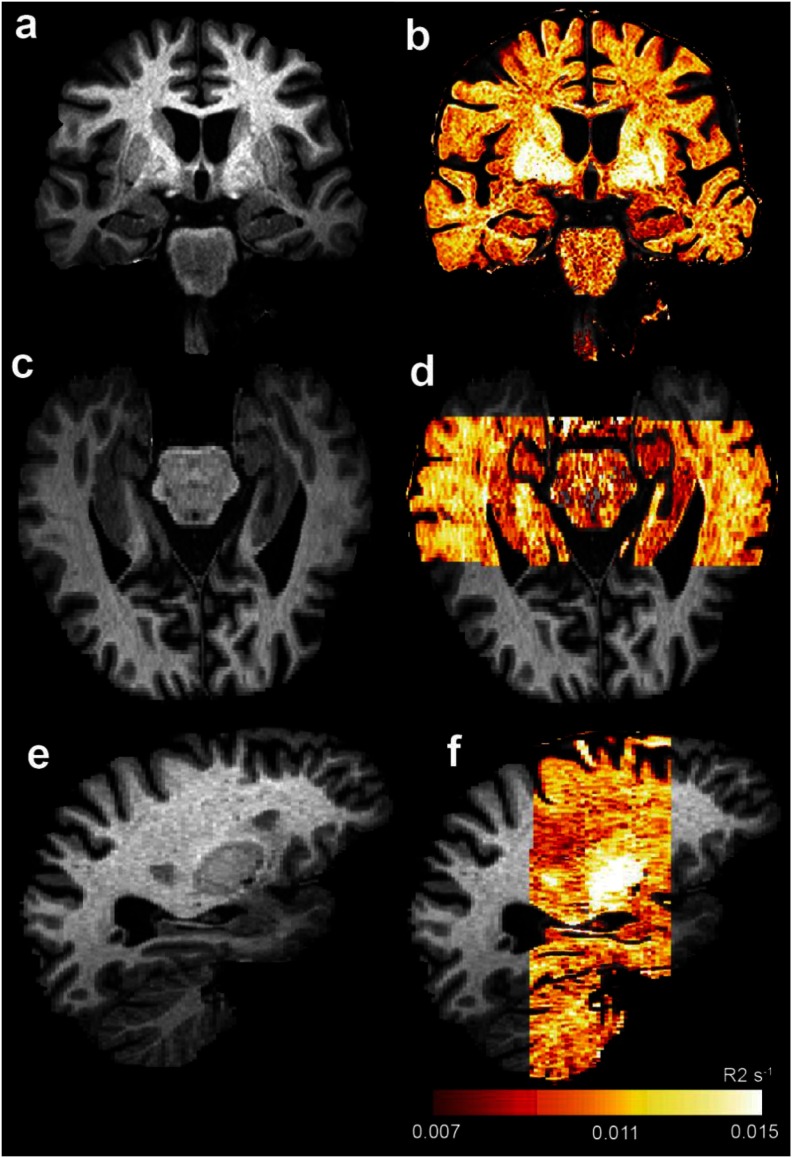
AD in the absence of hippocampal atrophy, but with hippocampal R2 reduction (T2 prolongation). Panels (a), (c) and (e) show coronal, axial and sagittal views of a T1-weighted MPRAGE scan. Panels (b), (d) and (f) show an R2 map (R2  =  1/ T2) overlaid onto this, demonstrating low R2 (high T2) in the hippocampus relative to cortical grey matter.
